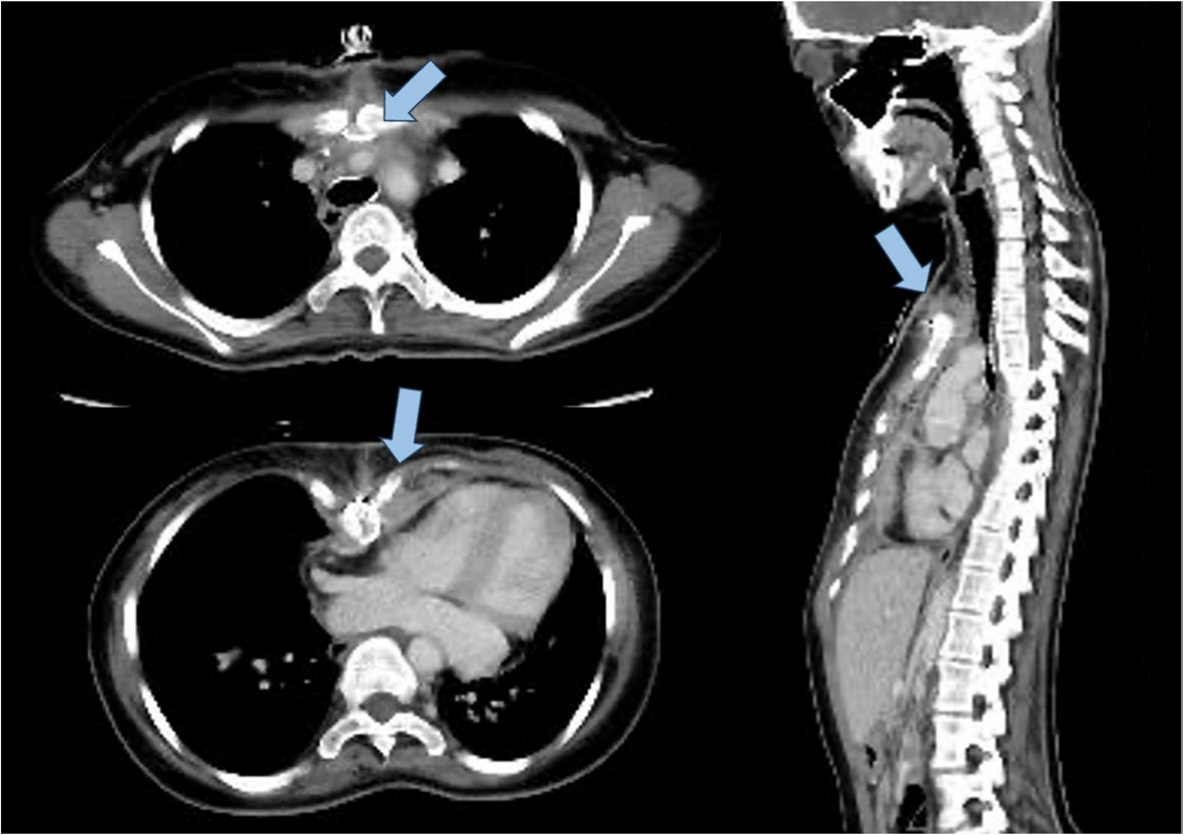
Computed tomography results (arrow; fluid collection)
